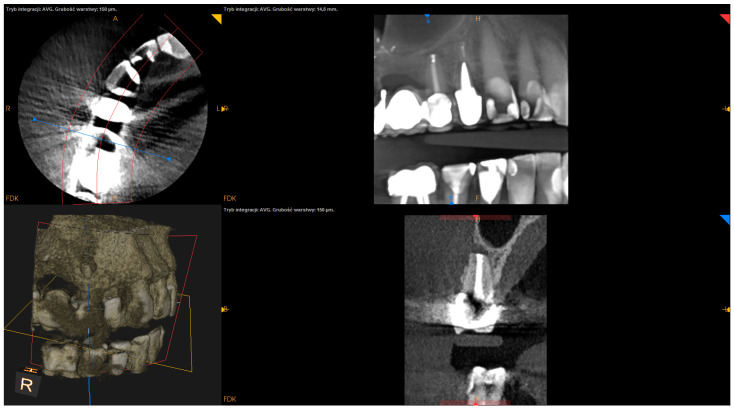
CBCT image (CS 8100 3D Carestream Dental^ⓡ^) example of uncertain healing based on PENN 3D criteria. Non-English annotations present software technicalities (AVG integration mode and layer thickness).
